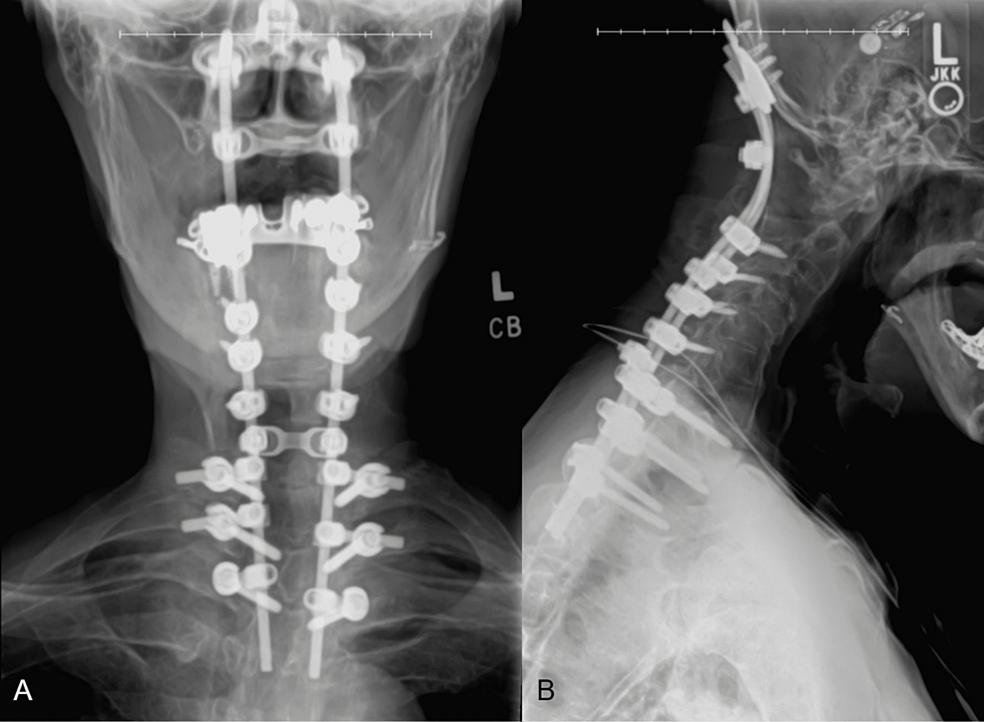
Coronal (A) and sagittal (B) views of postoperative CT scan of the posterior cervical fusion of occiput to T3 CT: computed tomography
